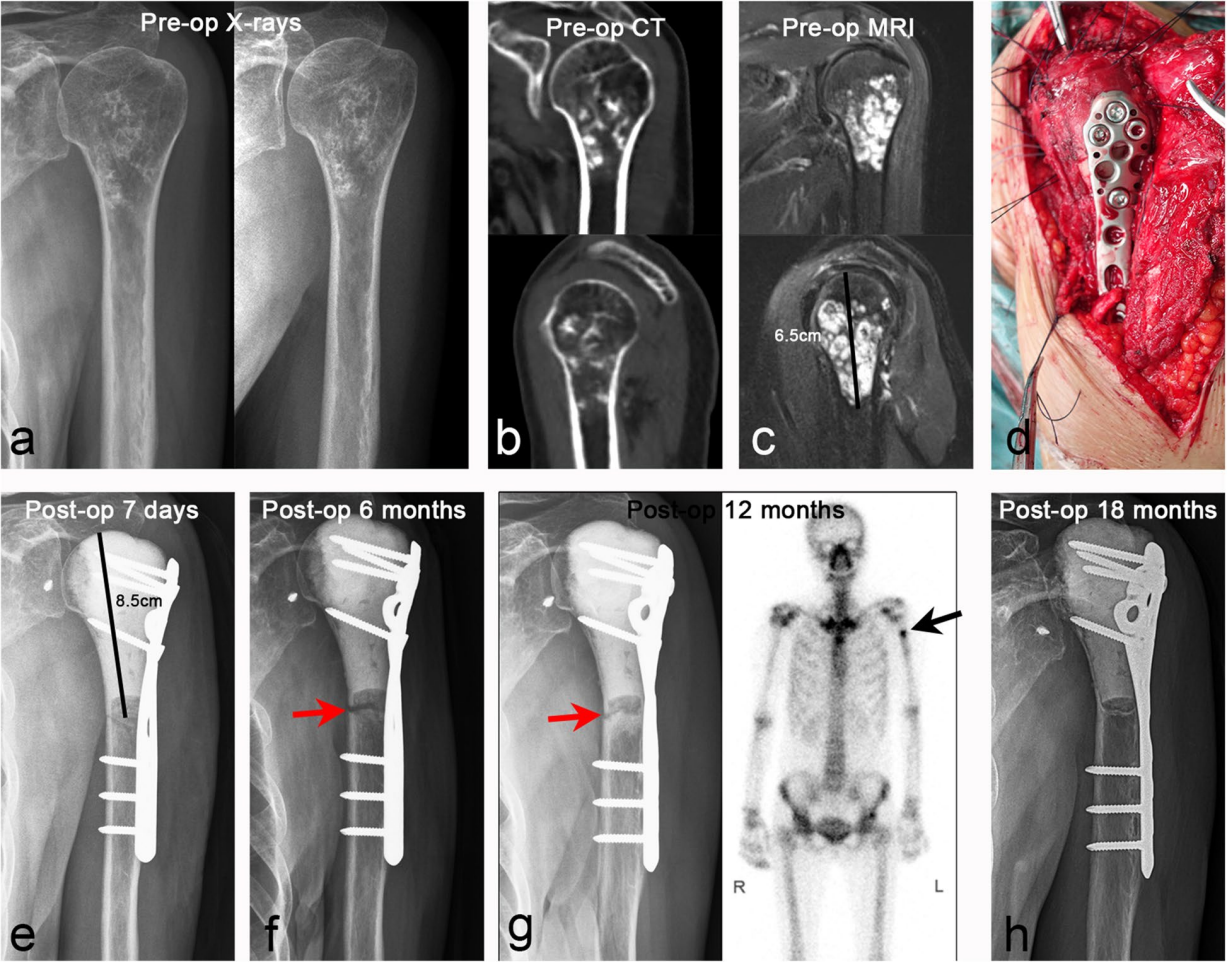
Case 18. A case of stage IIA chondrosarcoma in proximal humerus. a–c Anteroposterior and lateral X-ray, CT scan, and MRI images. d The allograft was fixed with a long segmental plate to repair the bone defect after tumor resection, and the rotator cuff and joint capsule were repaired. e–g The postoperative anteroposteric X-ray at 7 days, 6 months, and 12 months showed bone resorption of the host–donor junctions at 6 months after operation, and SPECT scan at 12 months showed active metabolism at the host–donor junctions, indicating that the process of bone healing was in progress. h The postoperative X-ray at 18 months showed that the host–donor junction was completely healed, and the internal fixation was firmly fixed
